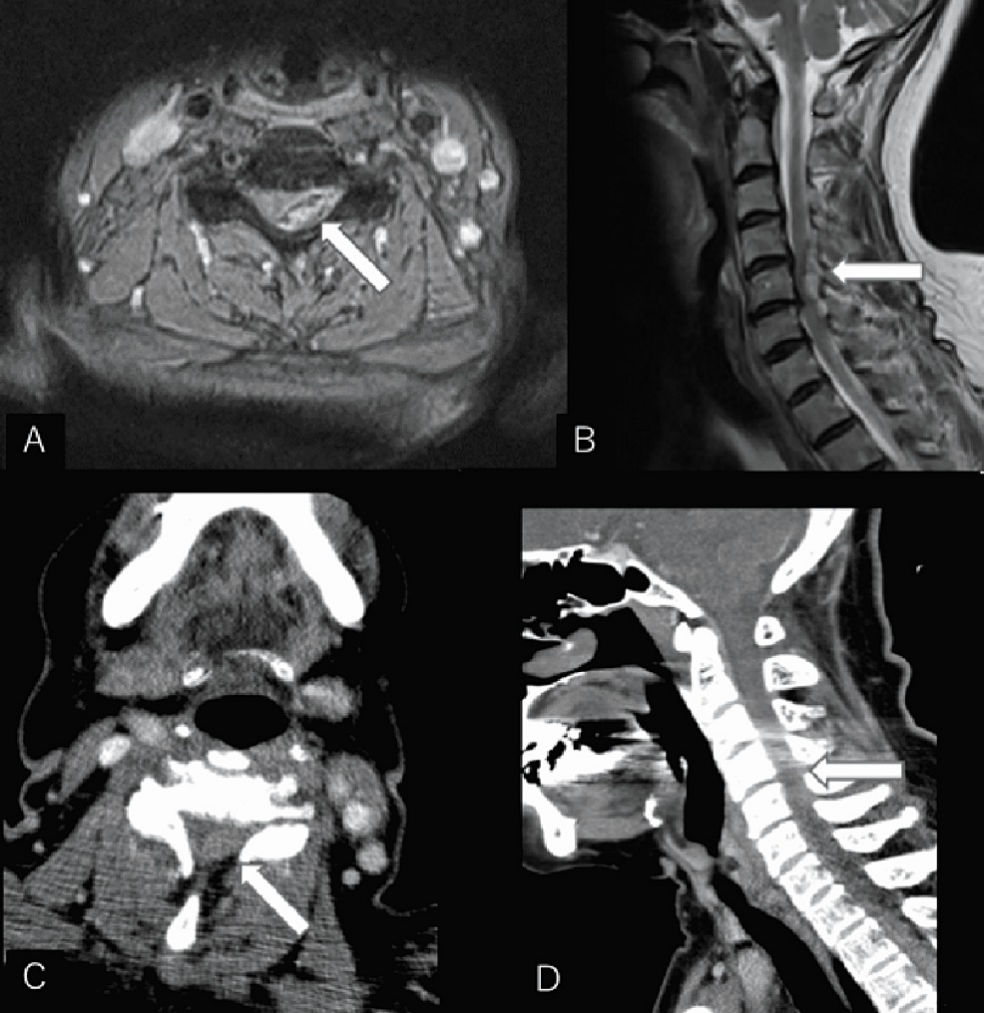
Case 1 preoperative images (A, B) Preoperative cervical T2-weighted MRI images at the C5 level in axial (A) and sagittal (B) views. (C,D) Preoperative cervical CT scan at the C5 level in axial (C) and sagittal (D) views. These show epidural hematoma on the left dorsal side at the C4-C6 level.
